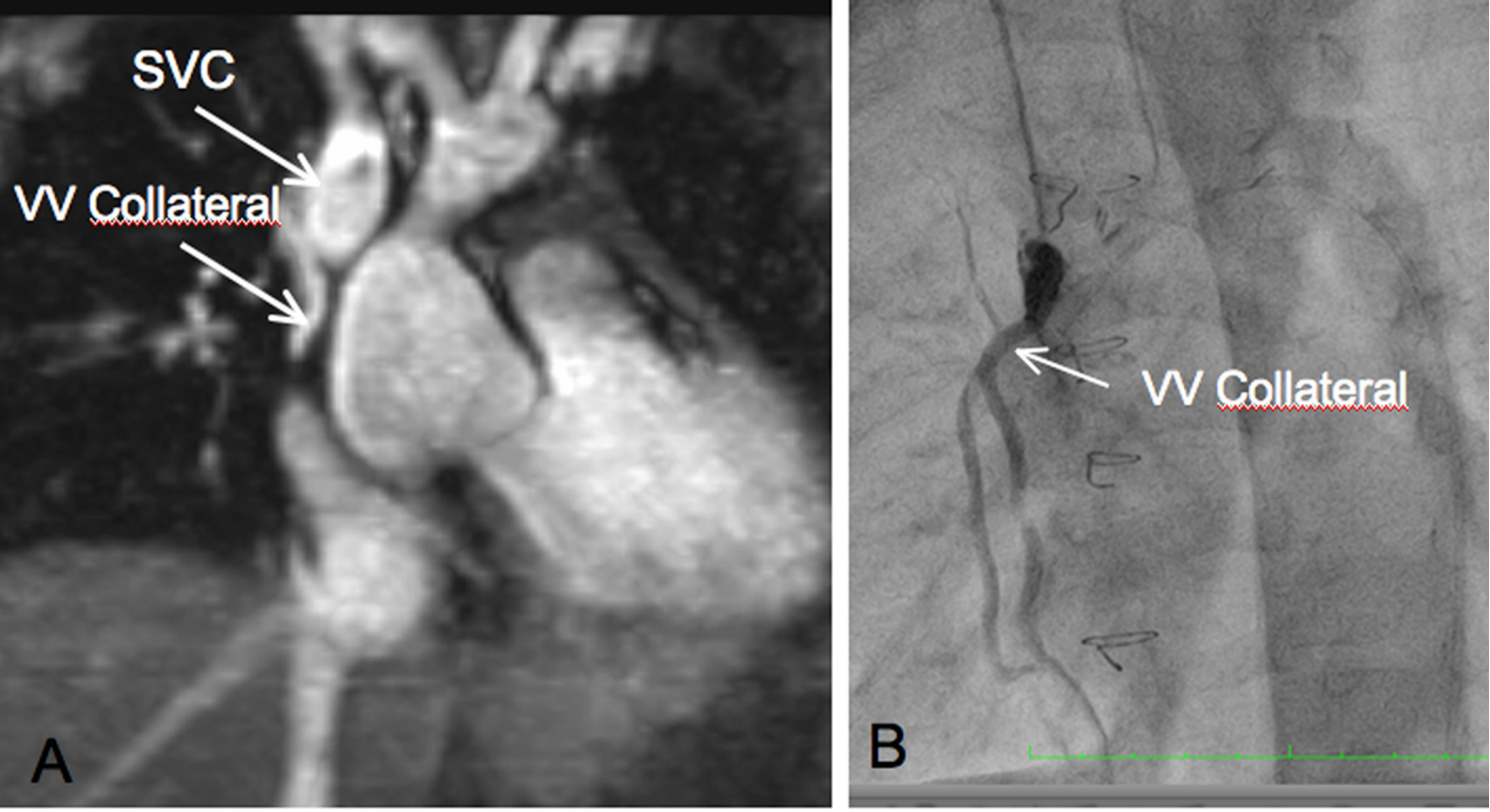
Veno-venous collateral in a patient candidate for Fontan. A: CMR: MIP reconstruction from TOF acquisition showing a veno-venous collateral from the SVC. B. Selective X-ray angiography in the veno-venous collateral.
